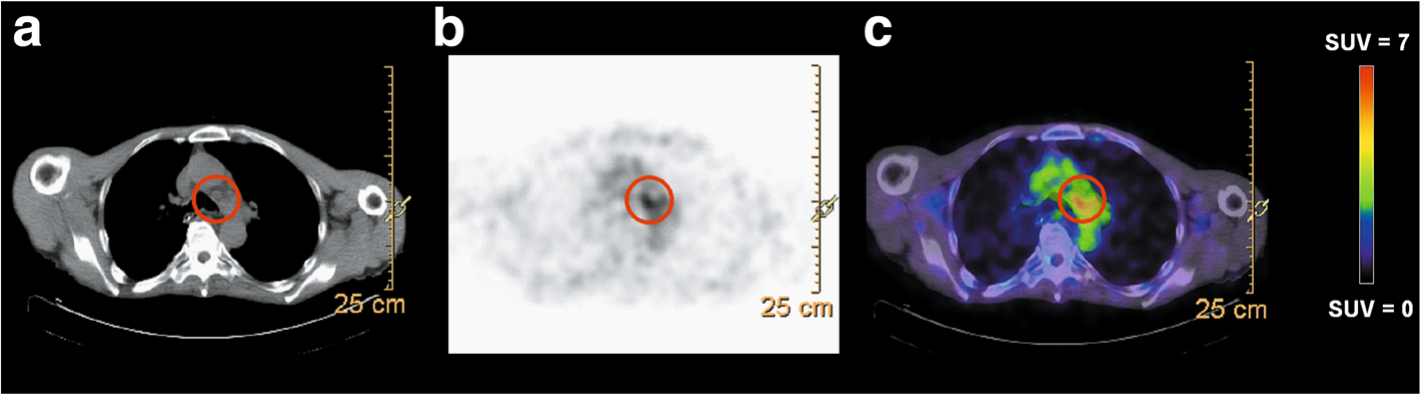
Example of tumour uptake of ^89^Zr-RG7356 at 96 h p.i.. Tumour lesion mediastinal/in the aorta–pulmonary window (patient 8, 450 mg cohort). a Low dose CT. b Attenuation-corrected PET. c Fused image
